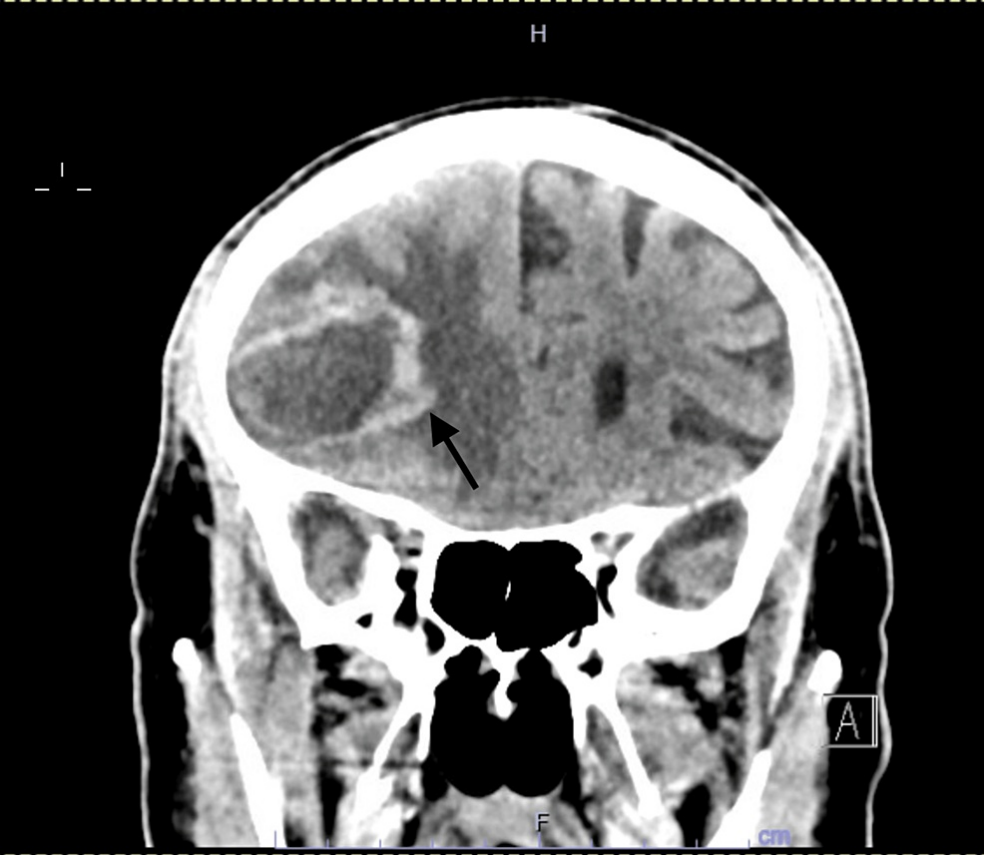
Coronal CT Head Coronal CT head showing the heterogenous right-sided mass (arrow)
